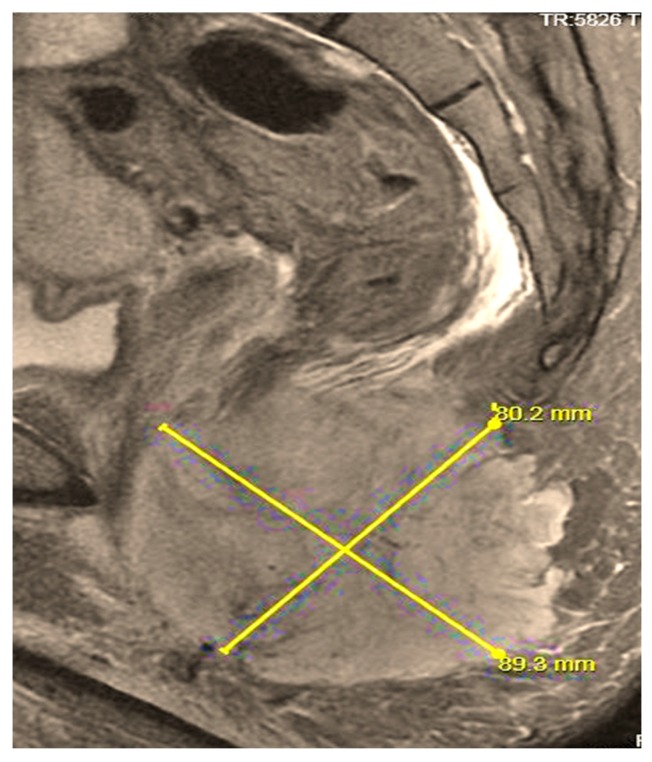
MRI (sagittal view) of 15 y/o patient with Stage IV perineal alveolar RMS.
